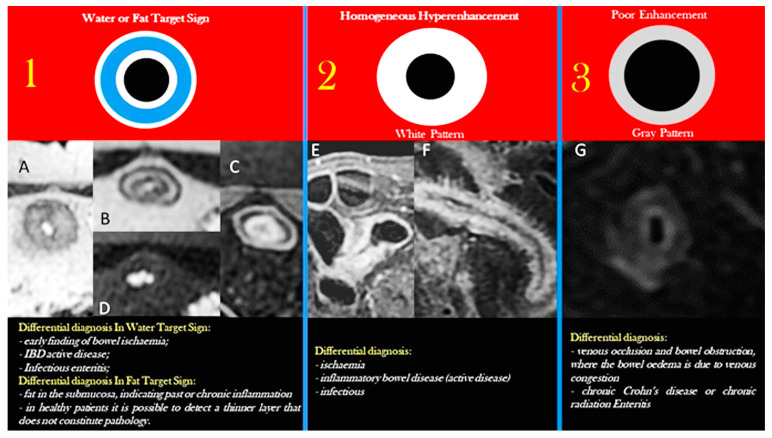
This scheme was borrowed from the enhancement patterns observable in CT enterography after the administration of an intravenous contrast medium: (1) target sign: indicates inflammation or ischemia of the bowel where the inner and outer high-enhancement layers correspond to the hyperemic mucosa and serosa, respectively, while the poor enhancement central layer represents the edematous or fat submucosa. (2) Homogeneous hyperenhancement or white pattern: global wall intense enhancement equal to or greater than that of venous vessels. (3) poor enhancement of the bowel wall is considered when the bowel wall is enhanced to be similar to that of the muscle. In 1A: the axial T2-weighted MRE image shows small-bowel wall thickening, mural edema (hyperintense mural signal intensity), and luminal narrowing (active Crohn’s disease phase). 1B,1D: the axial T2-weighted MRE image (1B) and fat-suppressed T2-weighted image (1D) show small bowel poor wall thickening and submucosa fat (hypointense mural signal intensity in fat-suppressed T2-weighted image) in the chronic Crohn’s disease phase. (1C) Axial contrast-enhanced fat-suppressed T1-weighted show stratified mural hyperenhancement. (2): coronal contrast-enhanced fat-suppressed T1-weighted MRE images (2E,F) show global small-bowel mural hyperenhancement. (3): poor enhancement. 3G: Crohn’s disease with active inflammation; axial contrast-enhanced fat-suppressed T1-weighted images show the resolution of the mural edema and hyperenhancement.
